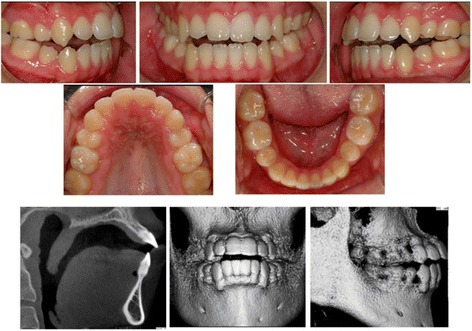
Result of orthodontic treatment in a high-angle patient. No bony issues can be detected clinically, but CBCT images show lower incisors with severe root resorption on a very thin mandibular anterior bony support
